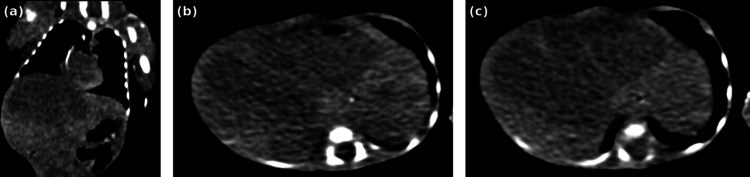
Contrast-enhanced CT demonstrating a multiloculated cystic hepatic mass with hemorrhagic features Contrast-enhanced CT images of the abdomen demonstrating a large multiloculated cystic hepatic lesion that occupies a significant portion of the liver parenchyma.
